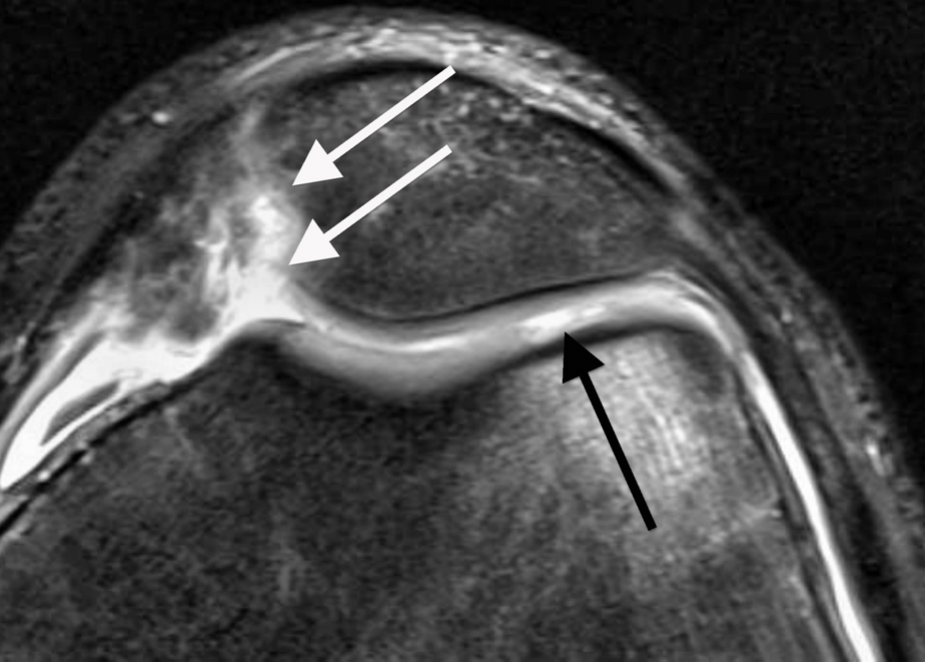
Axial PD-weighted TSE MRI of a 15-year-old male with a recurrent LPD. At the medial facet of the patella, a full-thickness defect of the cartilage (grade 4) with ulceration of the bone is demonstrated (white arrows). At the lateral femoral condyle, a cartilage composing > 50% of the cartilage thickness and showing a small ulceration to the subchondral bone is defined as a grade 3 lesion (black arrow).
